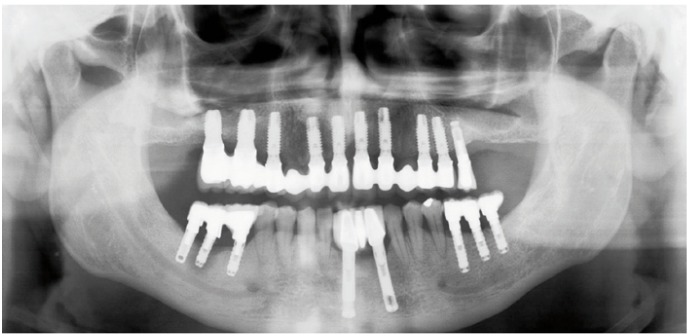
Case 2. 8. Panoramic X-ray view with the implants and prosthesis, one year after loading.
